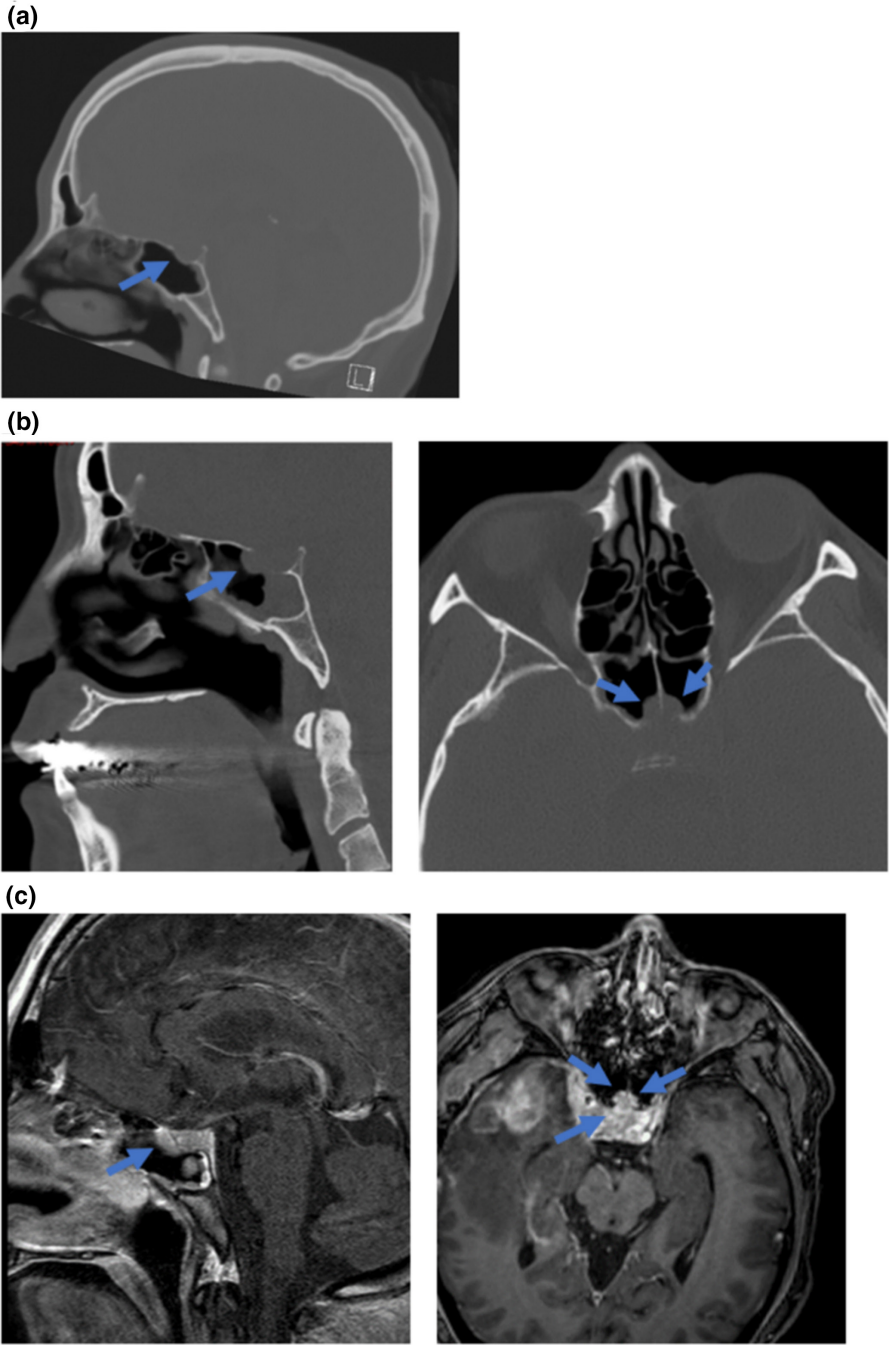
Pituitary expansion and erosion of the sella turcica. (a) Pre-operative CT in bone window demonstrates an intact anterior sella wall without evidence of dehiscence and pituitary herniation. (b) Post-operative day 6 CT sinus in bone window demonstrates a defect in the anterior wall of the sella with herniation of sellar contents into the bilateral sphenoid sinus. (c) Post-operative day 7 T1-weighted MRI images demonstrates a heterogeneously enhancing pituitary tissue herniating through anterior sellar defect into bilateral sphenoid sinuses.
